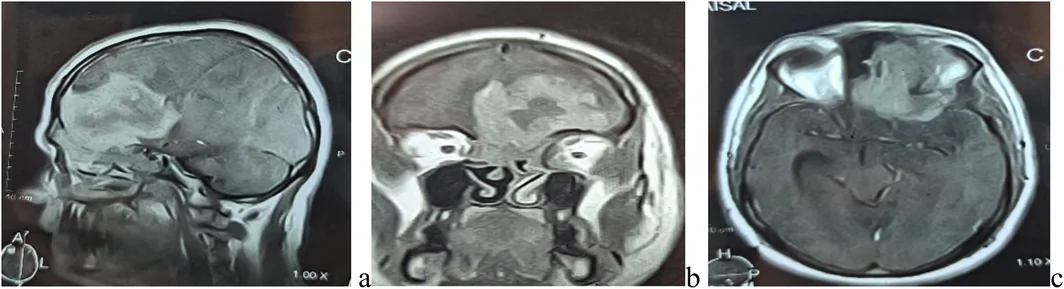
(a–c) Preoperative magnetic resonance imaging of the brain showing a well‐defined intra‐axial mass lesion in the left frontal lobe with surrounding vasogenic edema and mass effect, initially interpreted as a meningioma.
